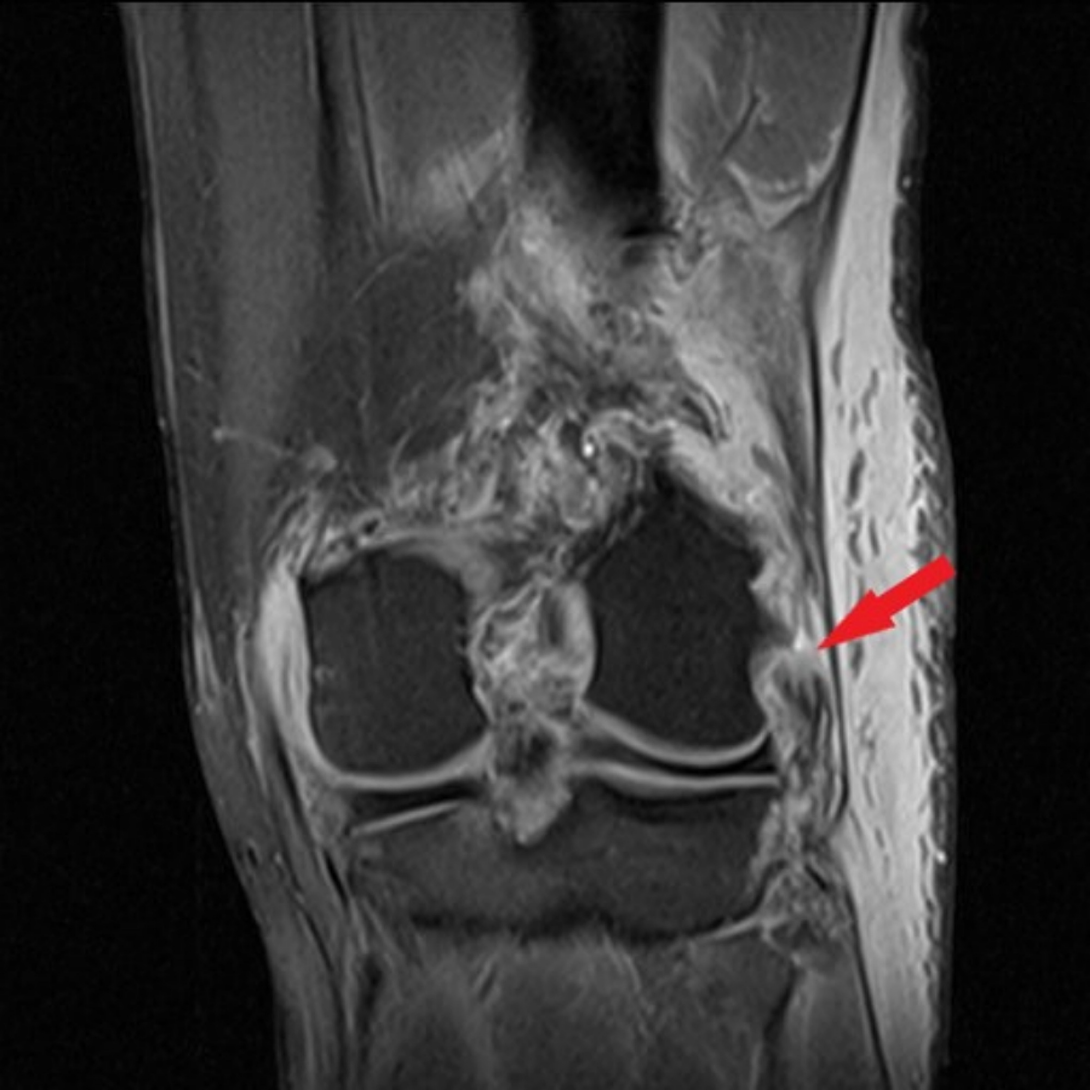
Coronal T2-weighted MRI of the left knee in patient 2 Coronal T2-weighted MRI of the left knee demonstrating rupture of the LCL (red arrow).
